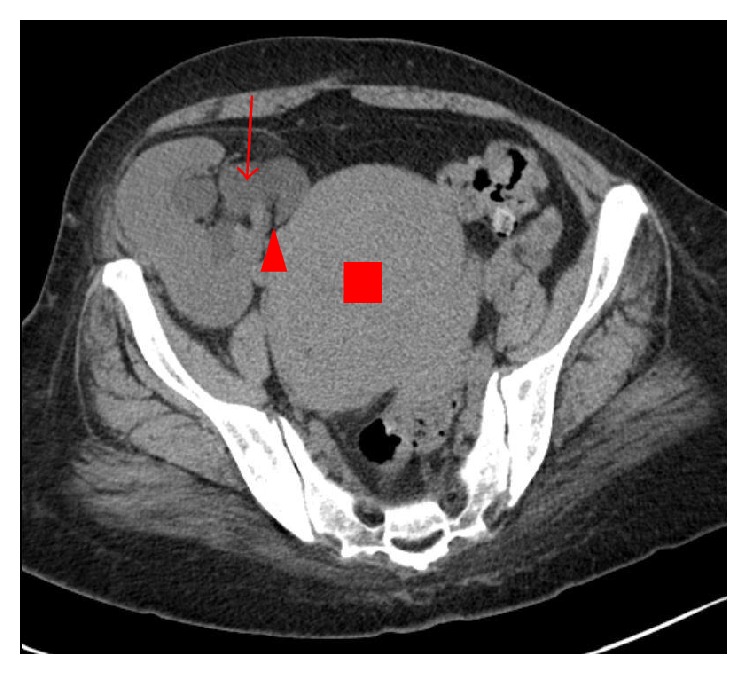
Plain computed tomography. A large uterine tumor (red square) compressed the distal ureter of the transplanted kidney (red arrowhead), causing hydronephrosis (red arrow).
